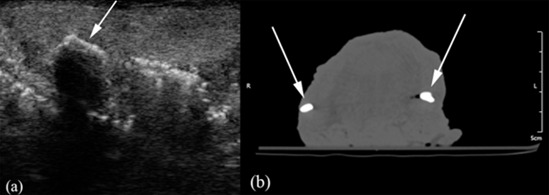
a: Arrow points to a stone foreign body on the ultrasound image. b: Arrows indicate two stone foreign bodies on the CT image.
